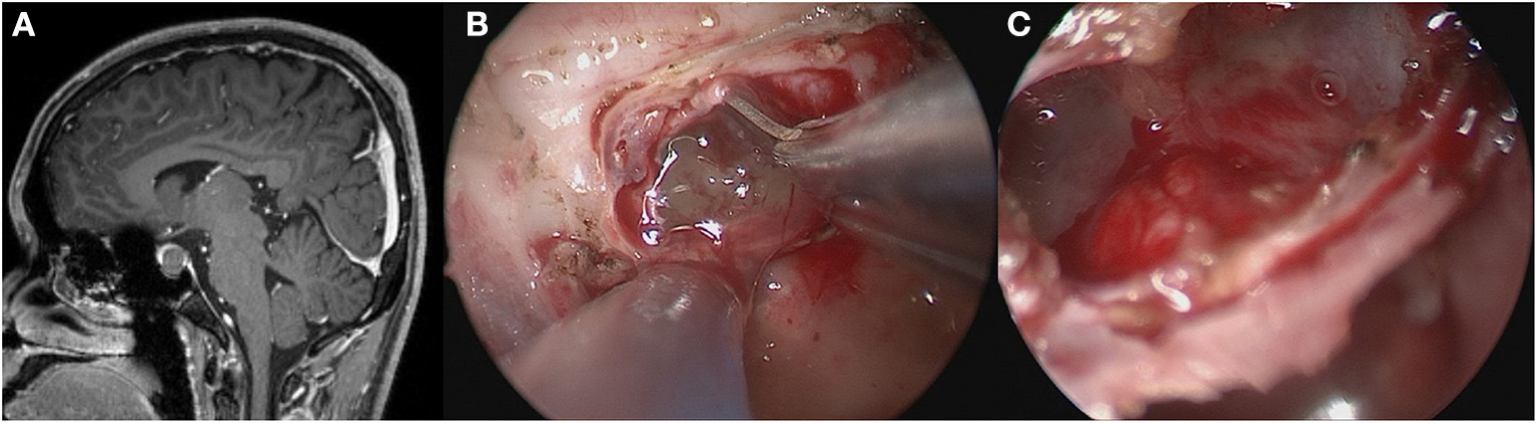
Partially resection of the cyst wall. (A) Preoperative contrast-enhanced MRI showed a cystic mass within the sella. (B) Exposed the gelatinous contents after opening the bottom of the saddle. (C) No further cleaning was done after the gelatinous contents were sucked out.
